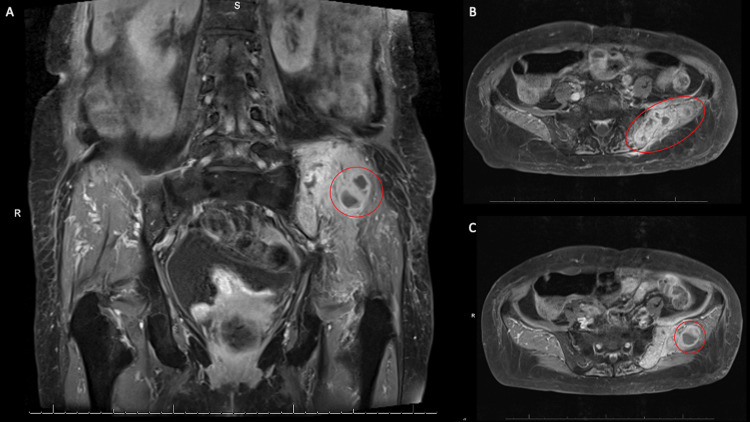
Pelvic MRI demonstrating osteomyelitis Red circles denote areas of interest. (A) The coronal section showing the infectious process on the left. (B) Axial section showing osteomyelitis of the left iliac bone. (C) Axial section showing left gluteal abscess. Radiology report described diffuse marrow replacement of the left posterior superior iliac spine extending into the left iliac wing with heterogeneous enhancement, central necrosis, and loss of cortical margins. This process appears to cross the sacroiliac joint with focal involvement of the subchondral left sacral ala and is favored to represent an osteomyelitis. Rim-enhancing soft tissue decompresses from the left iliac bone into the left gluteal soft tissues, left paraspinal musculature and subcutaneous soft tissues is favored to represent abscess formation. Mild edema and enhancement along the undersurface of the left iliacus muscle belly.
